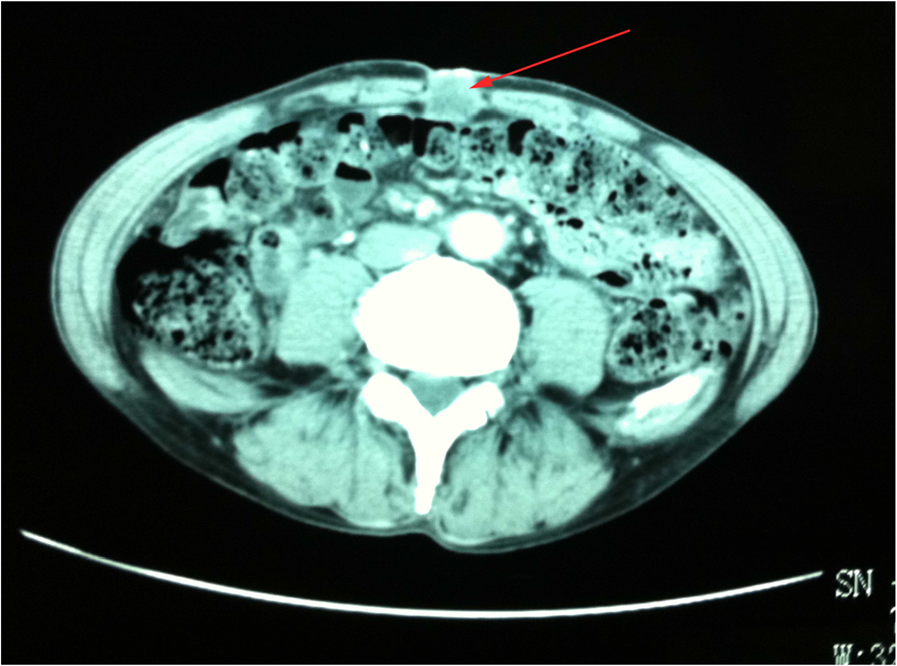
Epigastrium computed tomography: an ill-defined mass at the umbilical area shown by the red arrow.
